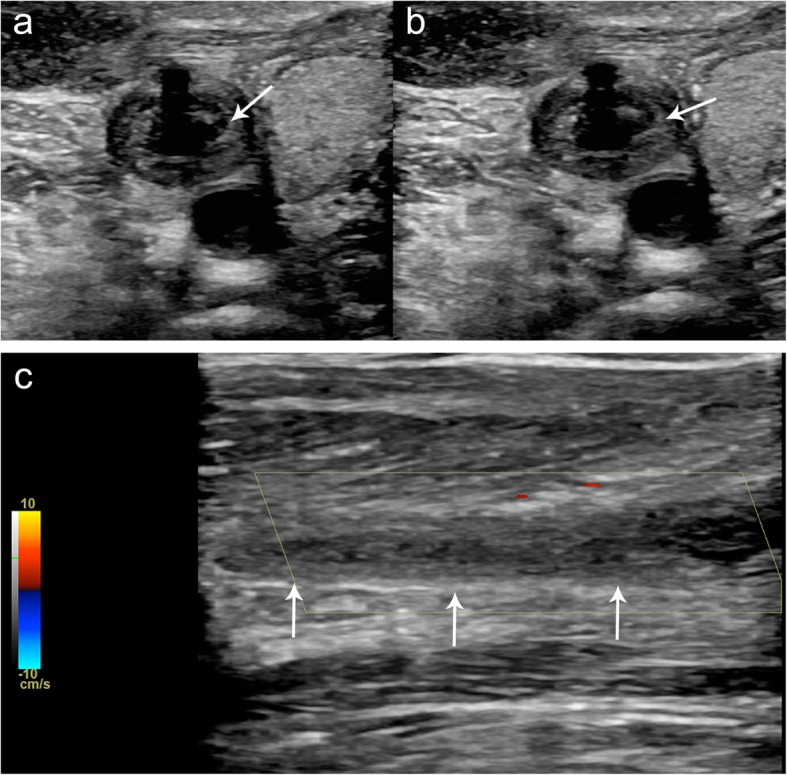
Lemierre’s syndrome. Contrast-enhanced axial (a) CT image of the thoracic inlet demonstrating a filling defect (asterisk) within the left internal jugular vein consistent with acute thrombus. Contrast-enhanced coronal (b) CT image demonstrates the extensive inflammatory changes and abscesses (arrow) within the soft tissues of the left neck. Together, these findings are consistent with the findings of Lemierre’s syndrome with extension into the thoracic inlet. Axial noncontrast CT (c) images in a companion case demonstrating multiple septic emboli within the pulmonary parenchyma (arrows). Note that a large right and small left complex pleural fluid collection (asterisk) was also present in this patient
